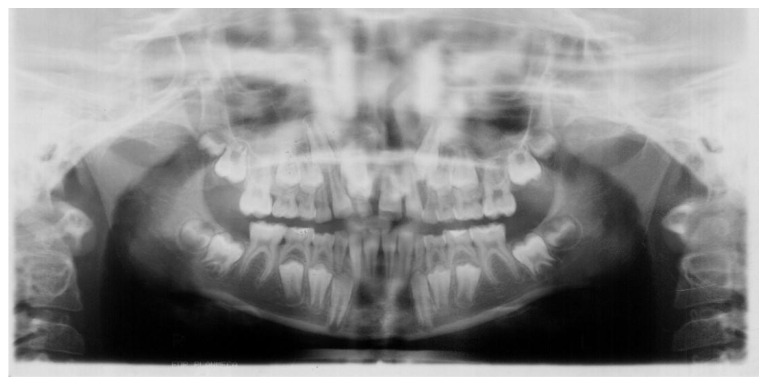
First panoramic radiograph, taken when patient was 9 years old, showing presence of all permanent teeth including third molars and one extra tooth. This supernumerary tooth was located between the cervices of the teeth 12 and 21, hindering eruption and development of the tooth 11. The distal angulation of both second lower premolars was also noticed. The alpha and beta angles of the tooth 13 are 16° and 3°.
